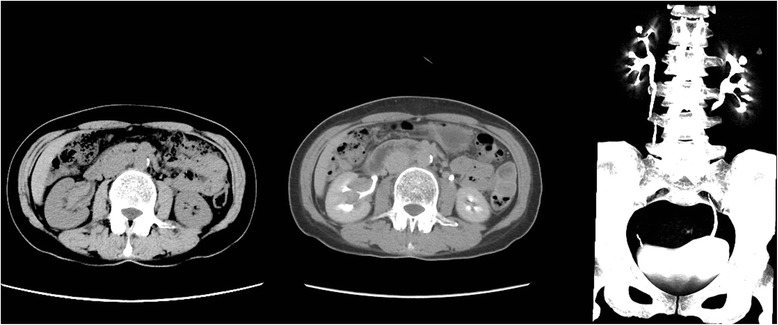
Abdominal computed tomography scans. These scans showed a soft-tissue density mass with an irregular border and mild homogeneous enhancement in the right renal pelvis and calyces
